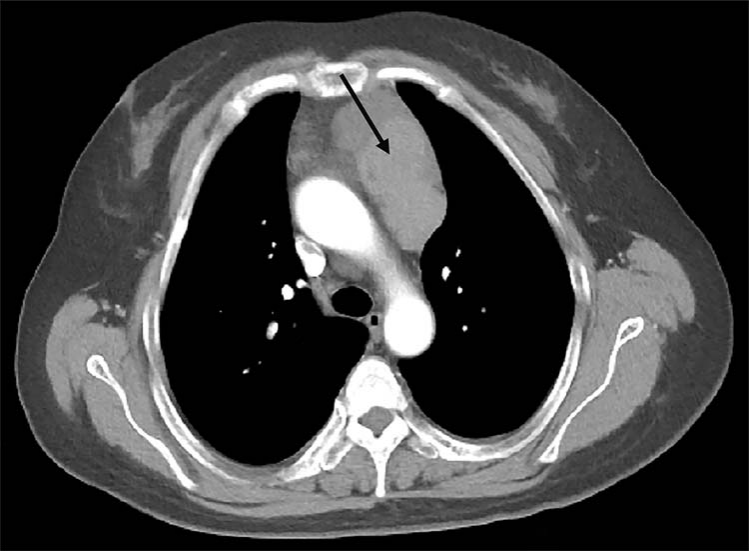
Enhanced CT scan of the chest revealed an anterior mediastinal tumor (black arrow).
